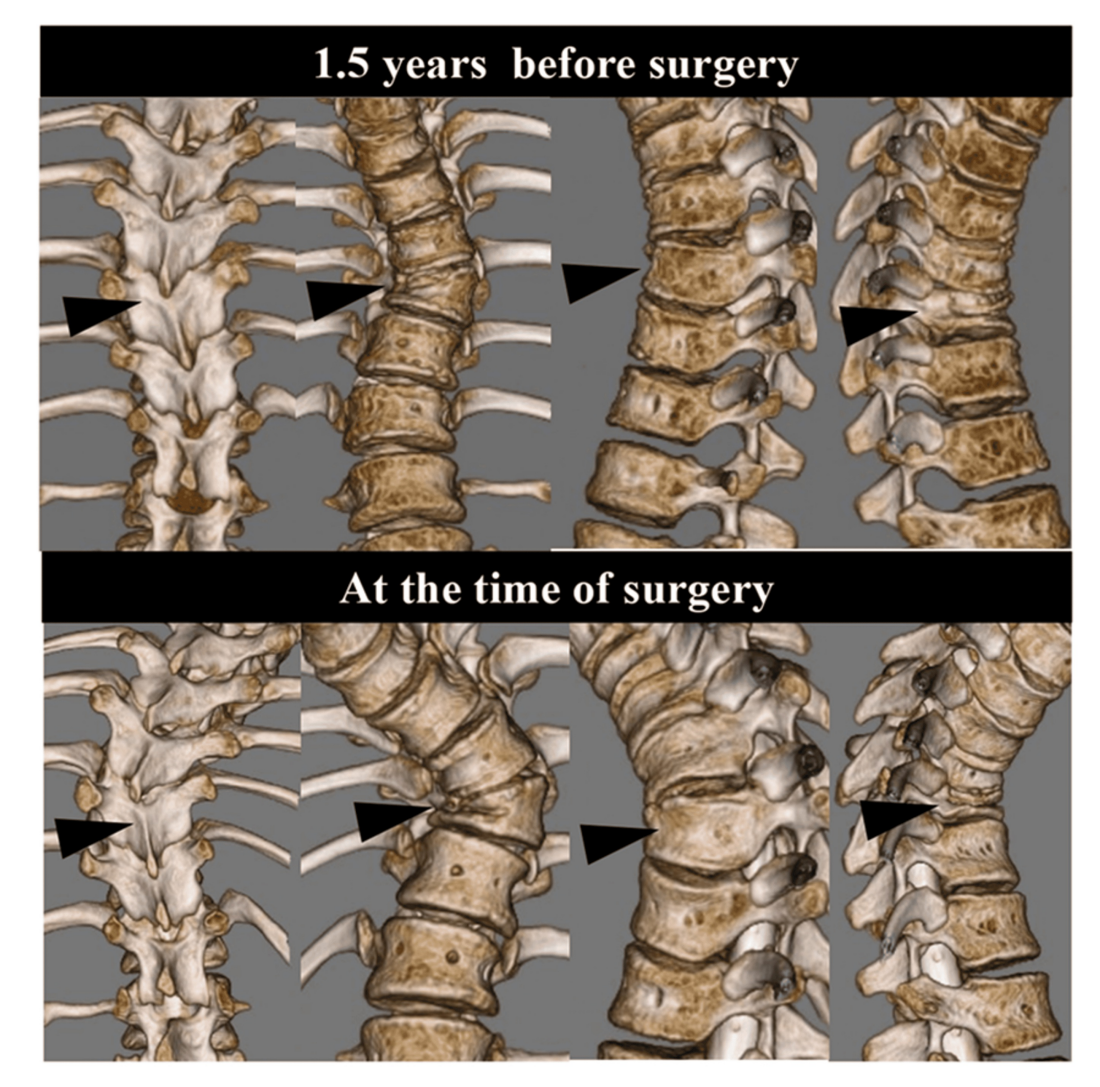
Three-dimensional computed tomographic (3DCT) images 1.5 years before and at the time of surgery The 3DCT images in the upper column were obtained 1.5 years before surgery, while the 3DCT images in the lower column were taken at the time of surgery. Both images were viewed from posterior, anterior, left, and right, respectively, from the left. The T10-wedged vertebra, indicated by the black triangle, exhibited a notable degree of deformation and remarkable progression of kyphoscoliosis during this interval.
